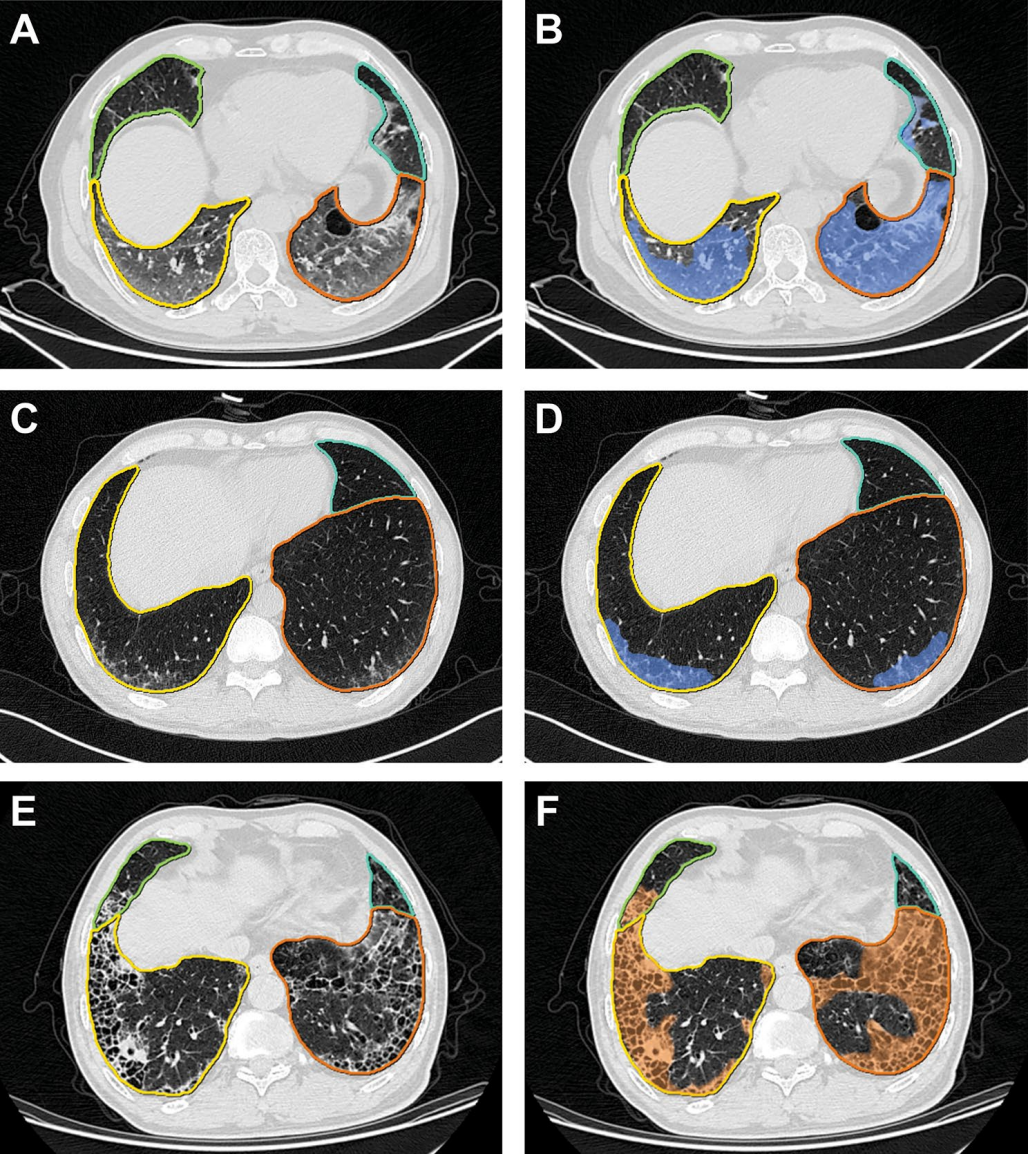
Examples of pulmonary lobe segmentation and opacity segmentation (A, B), pretreatment CT images of a patient with ground glass opacity, the patient developed grade 3 CIP at 133 days after ICI treatment. (C, D), pretreatment CT images of a patient with ground glass opacity, the patient developed grade 1 CIP at 118 days after ICI treatment. (E, F) pretreatment CT images of a patient with fibrosis, the patient did not develop CIP after ICI treatment
